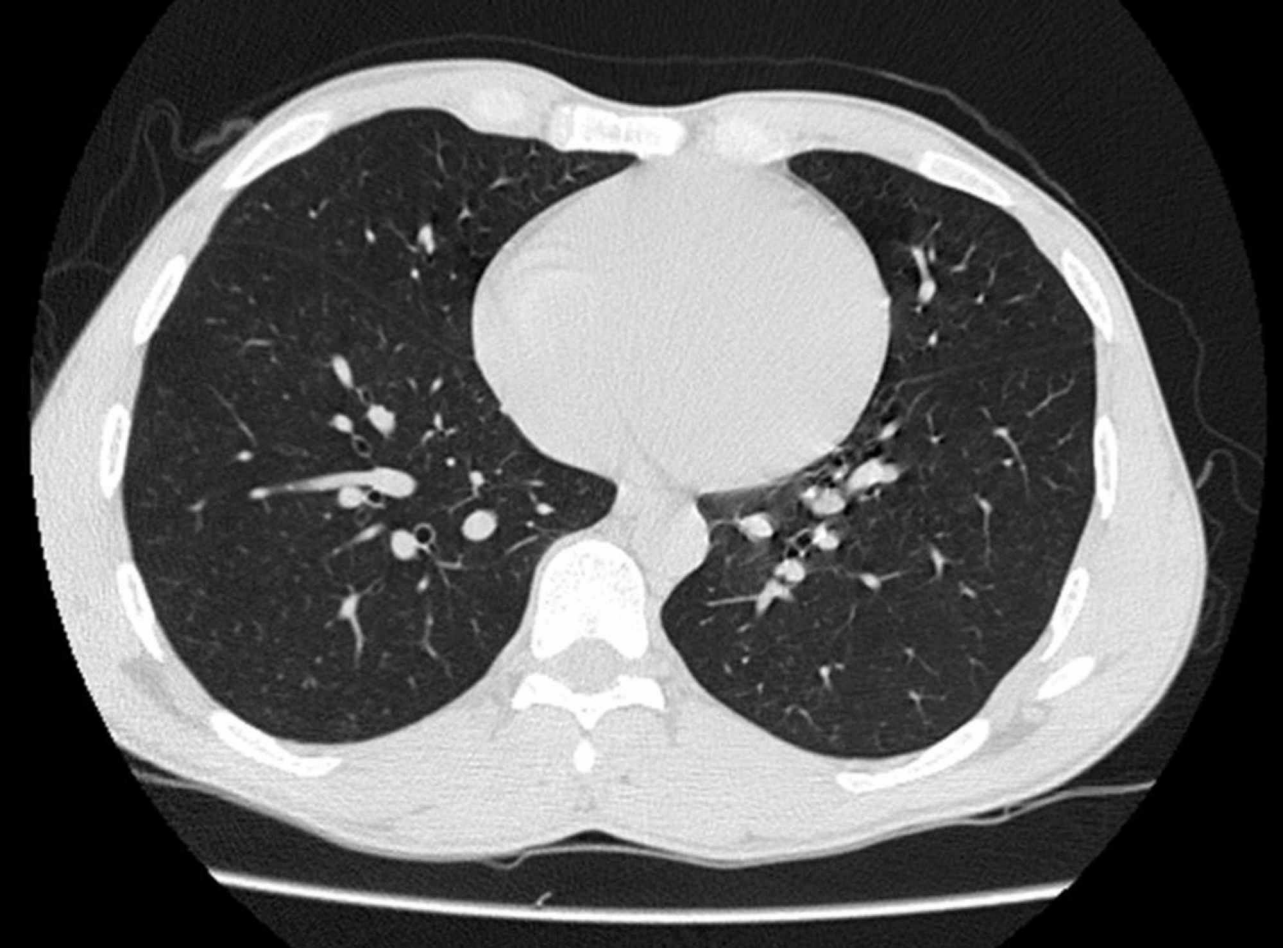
Axial image of high-resolution CT scan of chest in lung window at the level of heart reveals no abnormality in lung fields CT, computerized tomography
